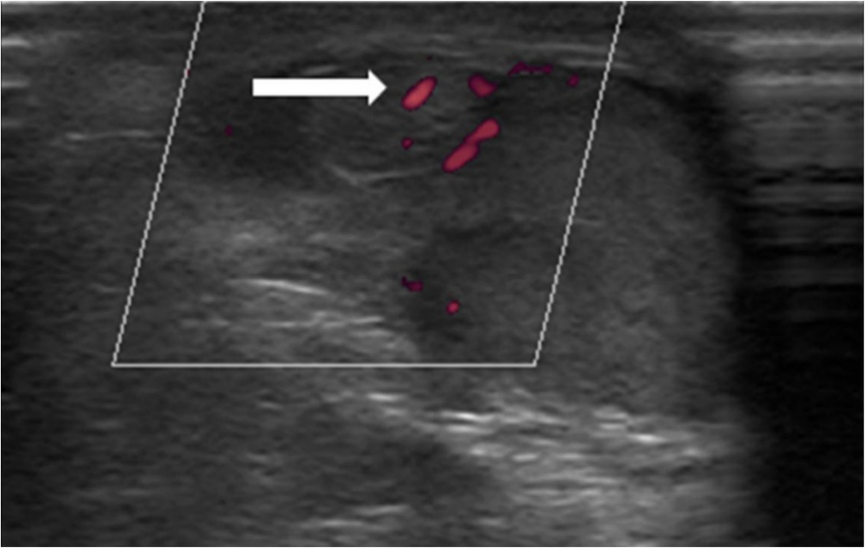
Power Doppler ultrasound of the mass in patient 2. Image shows normal flow (white arrow).
